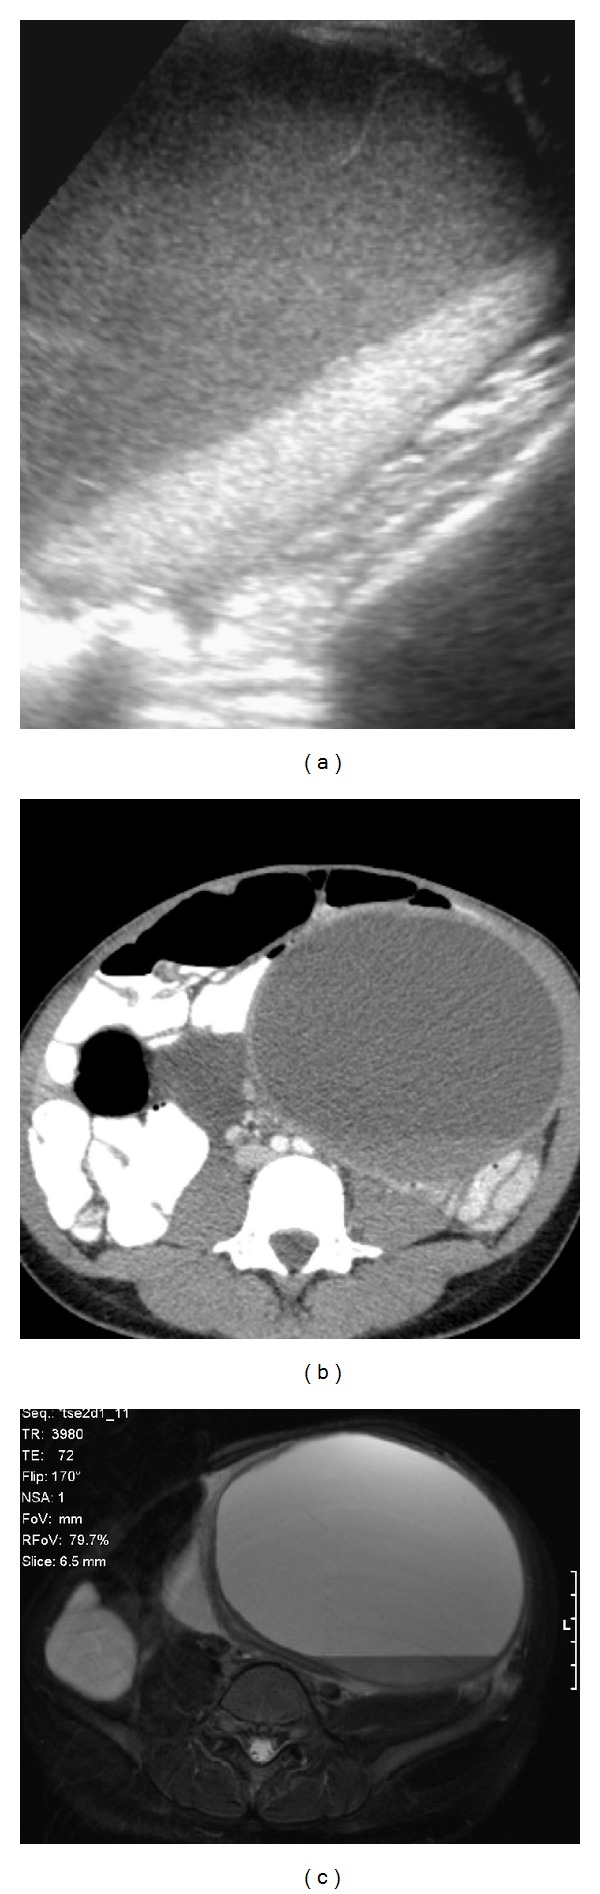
(a) Ultrasound demonstrating a complex cystic mass in the left adnexa with a blood fluid level and internal septations. (b) Axial CT demonstrating a large multiloculated left lower abdominal cystic “mass” with a blood fluid level. (c) Axial T2 MR image demonstrating a blood fluid level in a cystic “mass” in the left quadrant. There is also an additional component in the right lower quadrant.
